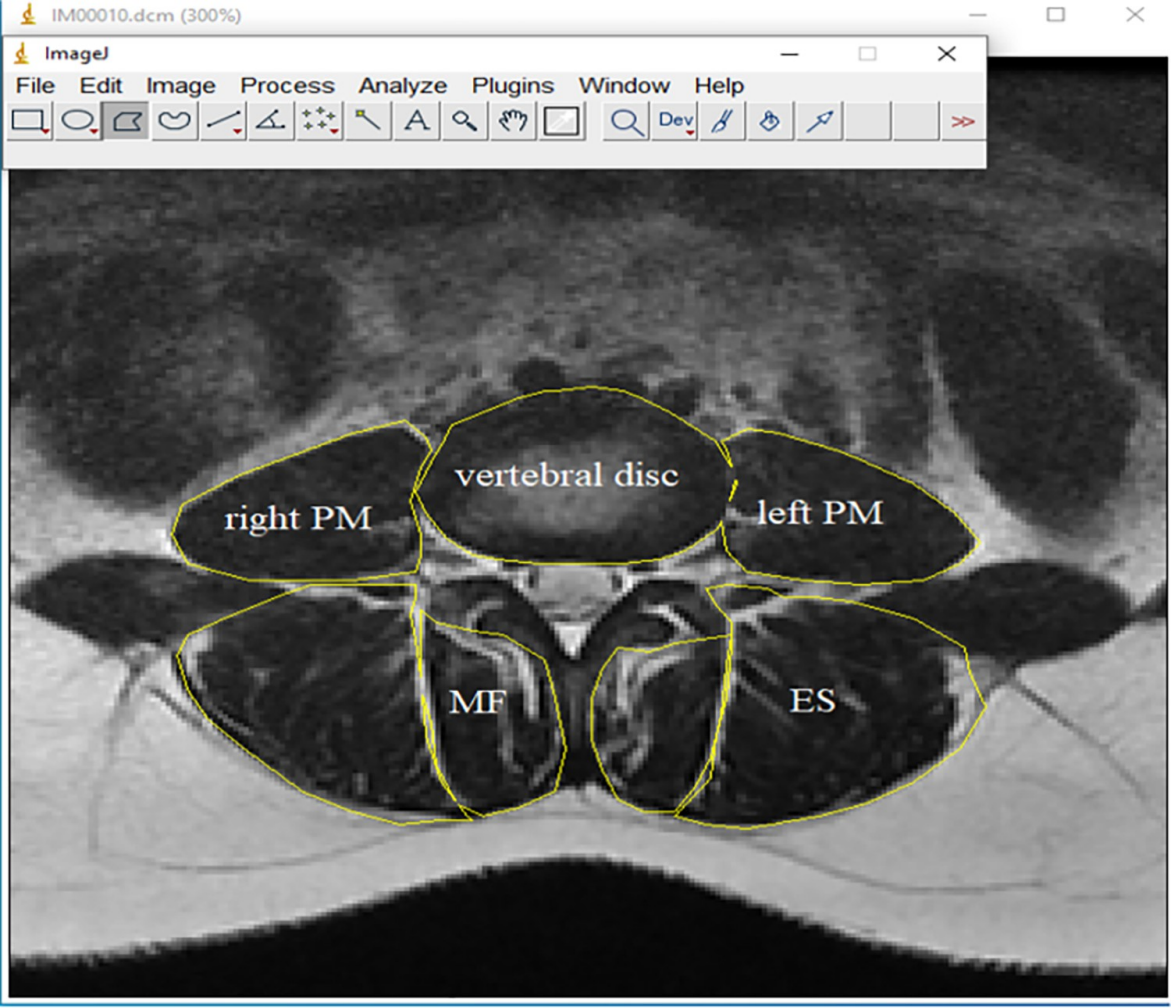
MRI of the lumbar spine in axial view at L4–L5 lumbar level.
